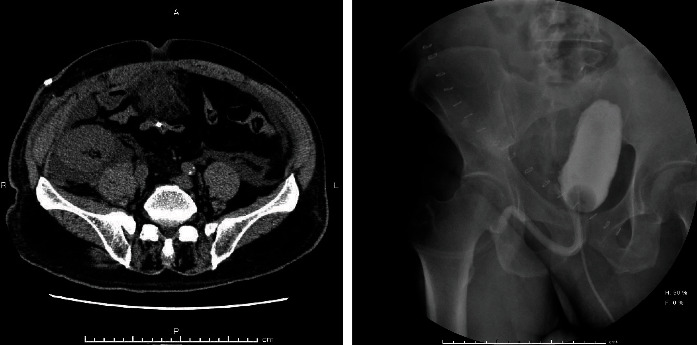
On postoperative day 5, fluid collection around the graft kidney is detected on computed tomography. Further, this fluid is not derived from the rupture of the anastomosis. Cystography reveals no leakage from the anastomosis.
